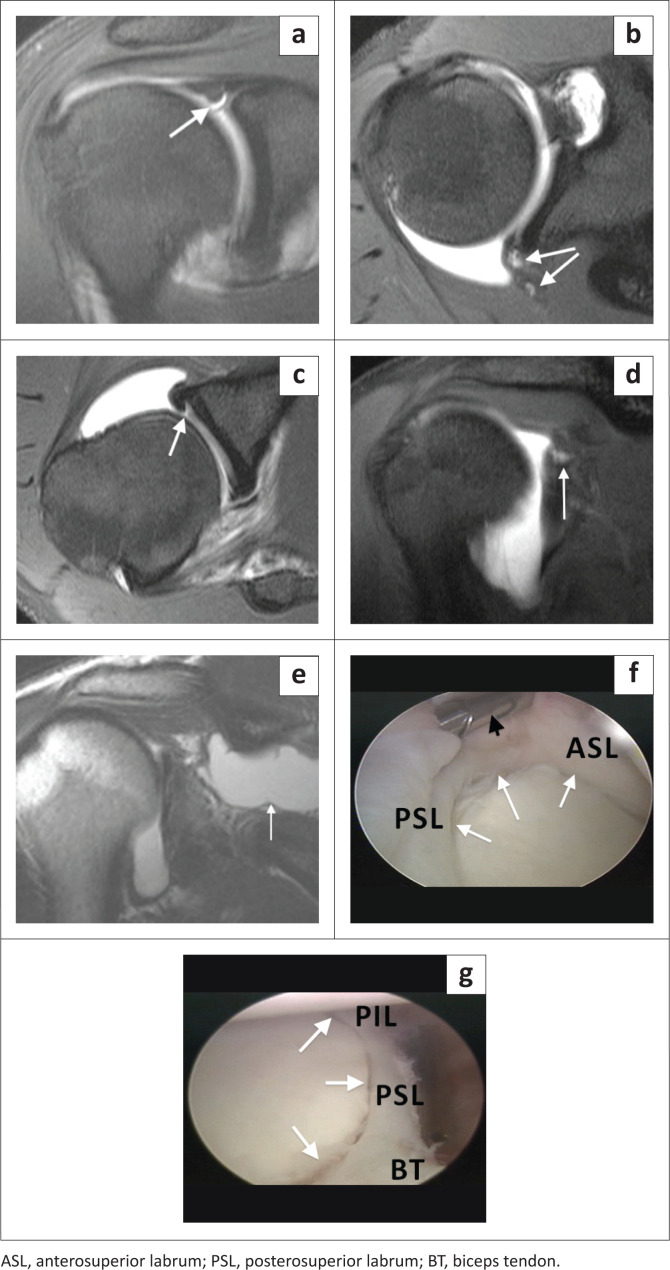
A SLAP type VIII lesion. (a) FS T1-weighted (T1W) MR arthrogram coronal oblique image demonstrating a superior labral tear (white arrow). (b) FS T1W MR arthrogram axial image depicting a tear of the posterior labrum with gadolinium-based contrast filling of the paralabral cysts (white arrows). (c) FS T1W MR arthrogram axial image at the level of the inferior margin of the subscapularis tendon showing the inferior extent of the tear of the posterior labrum (white arrow). (d) FS T1W MR arthrogram coronal oblique image demonstrating a paralabral cyst filling with only a very small amount of gadolinium-based contrast (white arrow). (e) T2-weighted (T2W) coronal oblique image depicting the true size of the paralabral cyst, highlighting the importance of some form of T2W sequence as part of the imaging protocol. (f) Arthroscopic confirmation of a SLAP VIII tear. White arrows indicate the superior labral tear. Black arrow indicates the arthroscopic probe overlying the biceps tendon. (g) Arthroscopic image demonstrating the posterior extension of the tear (white arrows) to involve the posterosuperior (PSL) and posteroinferior labrum (PIL).
